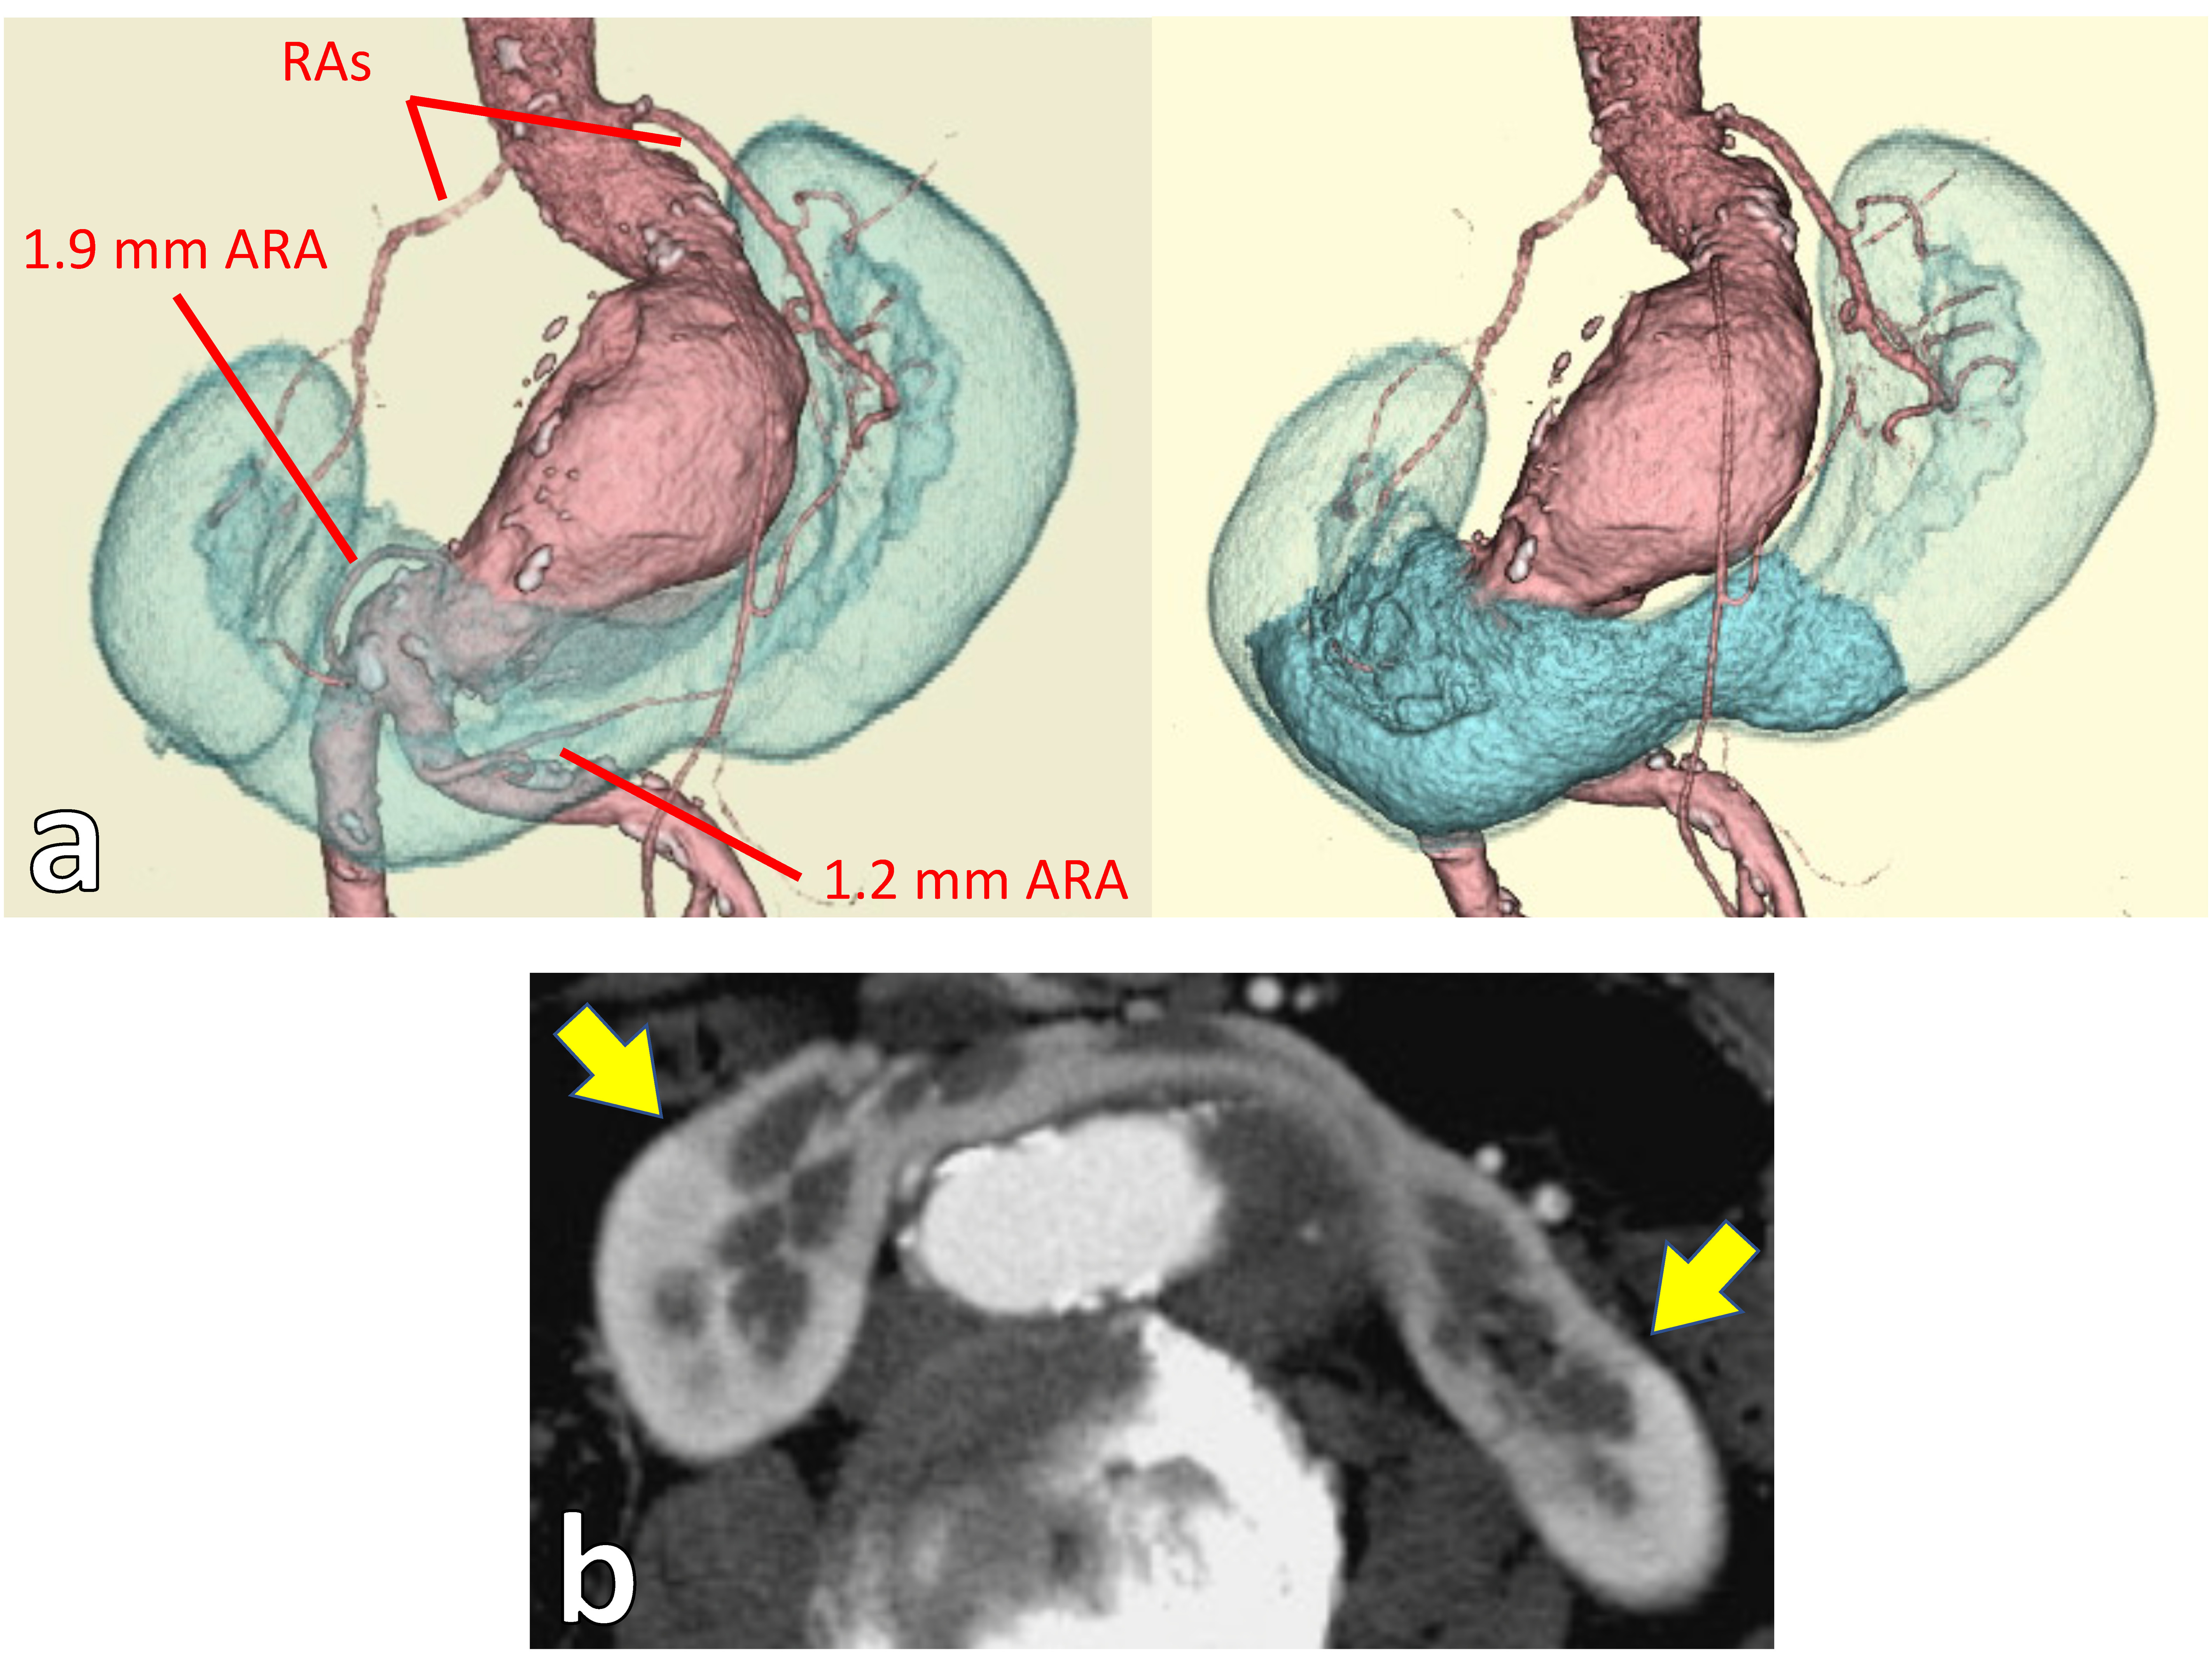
Fig. 1 Preoperative contrast-enhanced computed tomography and volumetry of the isthmus. (a) Preoperative three-dimensional reconstruction shows an infrarenal abdominal aortic aneurysm with accessory renal arteries originating from the aortic aneurysm and left common iliac artery. The deep blue area is the isthmus, which is between watershed lines, and it is supplied by accessory renal arteries. RAs: renal arteries; ARA: accessory renal artery. (b) Preoperative contrast-enhanced computed tomography shows clear watershed lines (arrows) at the margin of the isthmus. The calculated volume of the isthmus was 24.5% (94.15 cm^3^) of the total parenchyma (384.87 cm^3^).
